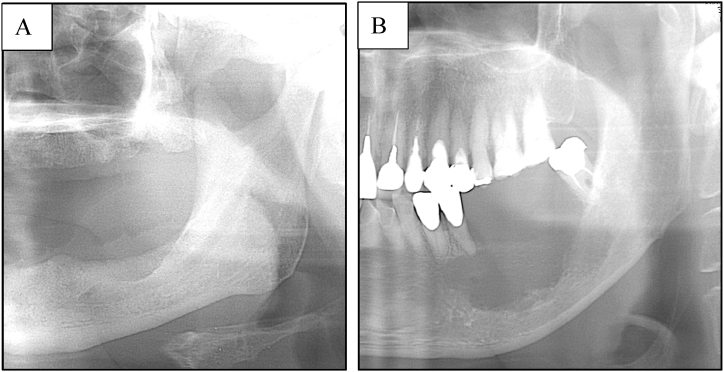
The radiographical type of mandibular resorption. A: pressure-type, B: moth-eaten type.
